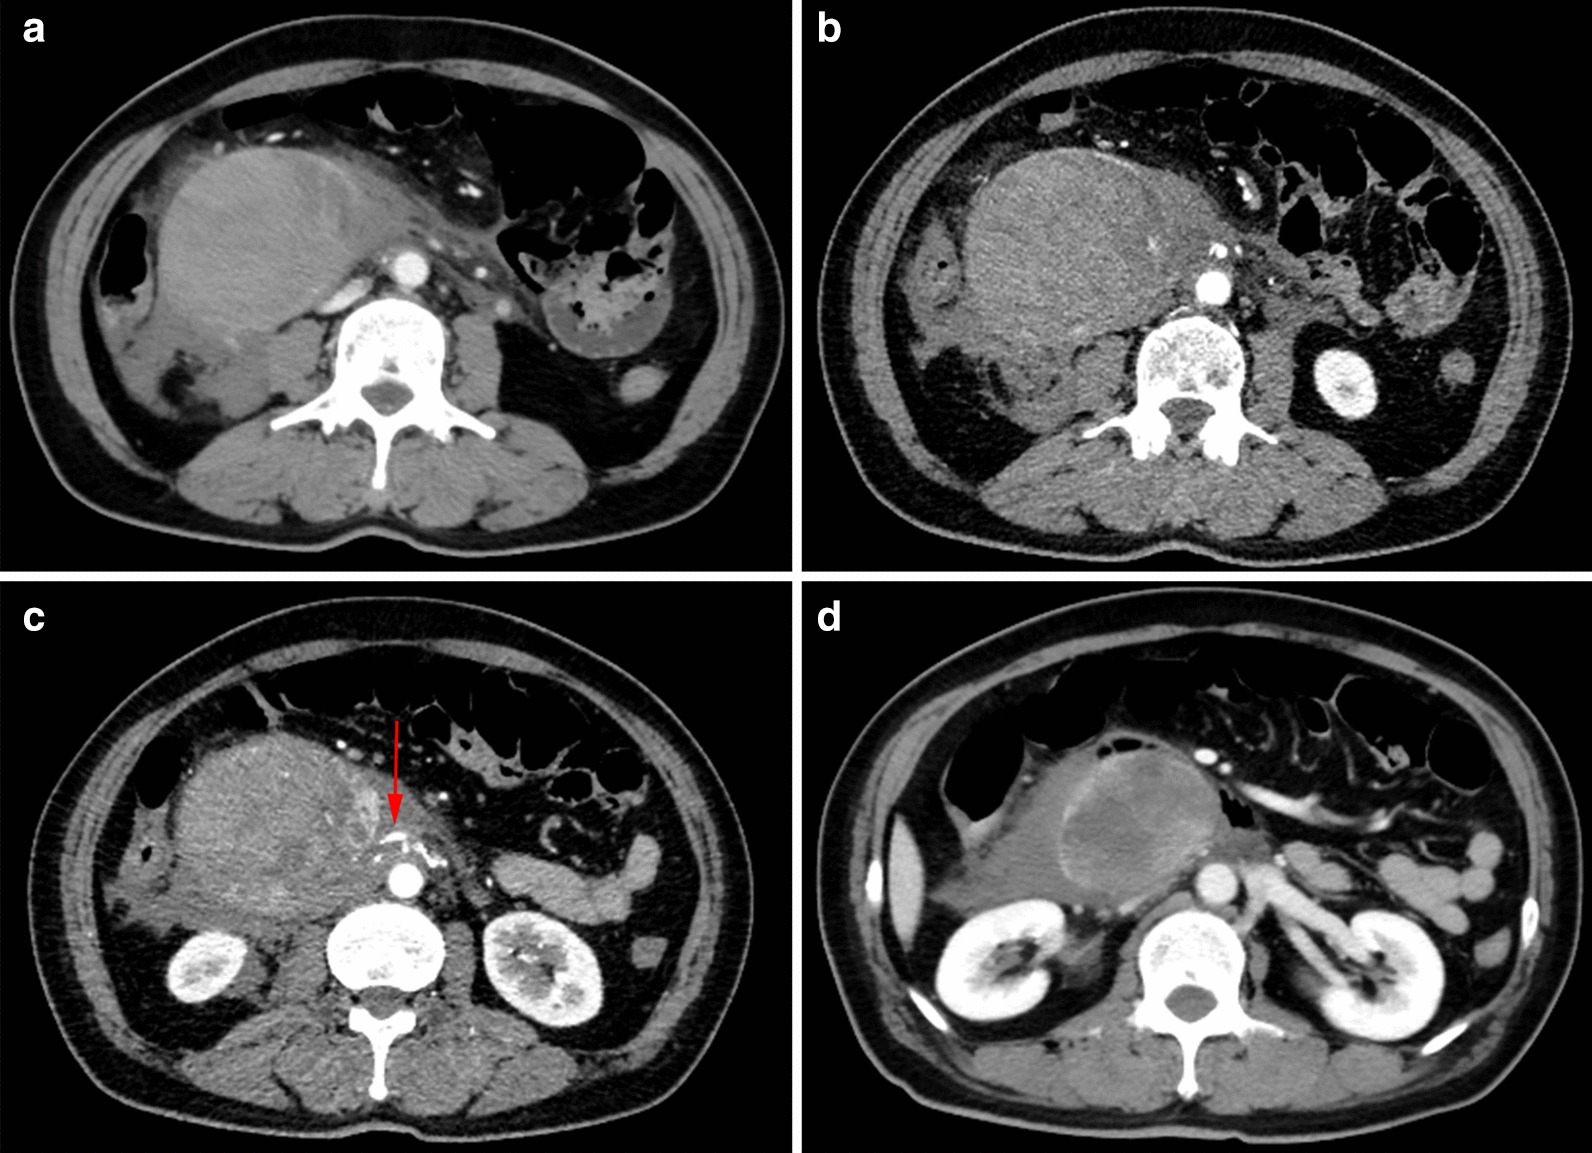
Computed Tomography Angiography (CTA) of superior mesenteric artery. The enhanced CT scan of the abdomen showed a retroperitoneal mass located posterior to the 3^rd^ part of duodenum and below the head of the pancreas. The retroperitoneal mass had a slightly increased but non-uniform density shadow at venous phase (a). No enhancement appearances at arterial phase (b). The abdominal aorta sent out small branches to supply the tumor (arrow) (c). There was plentiful effusion around the tumor (d)
